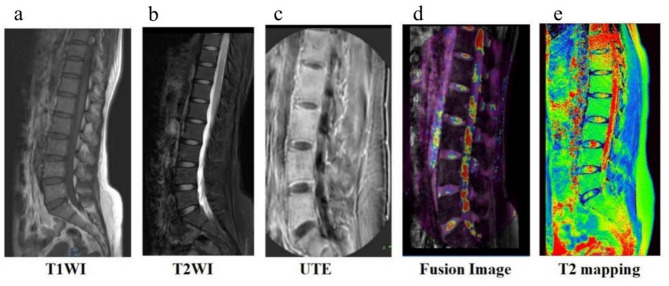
Magnetic resonance images of different sequences. From a woman who underwent a lumbar MRI examination, 28 years old, no history of low back pain, a. T1WI sequence image, b. T2WI sequence image, c. UTE sequence echo 1 image, d. UTE subtraction sequence and 3D water sequence overlay image, e. T2 mapping sequence image
